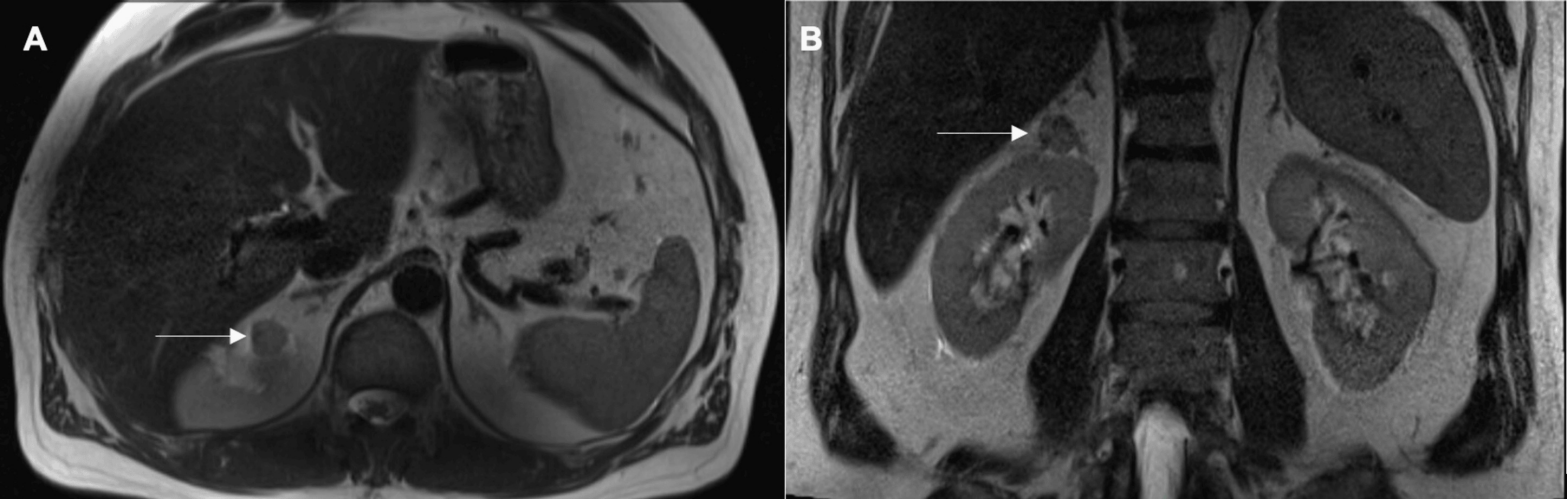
MRI of right renal mass. (A) Axial MRI demonstrating a 1.7cm exophytic lesion arising from the right superior renal pole (white arrow). (B) Coronal MRI demonstrating the same lesion.
